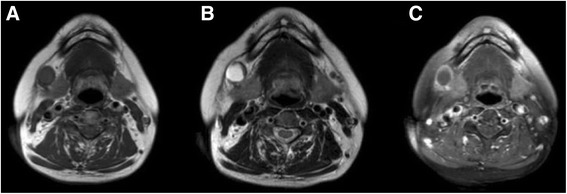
Preoperative MRI of the neck: a well-defined round mass proximal to the anterolateral side of the right submandibular gland, a T_1_WI revealed a well-distributed intermediate signal; b T_2_WI revealed a high signal; and c contrast-enhanced T_1_WI revealed an obvious enhancement of the peripheral wall
